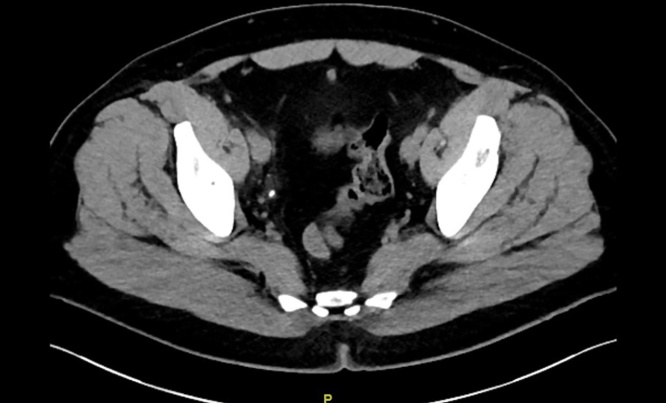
Abdominal computerized tomography (CT) scan revealed an 8-mm right distal ureteral stone.
